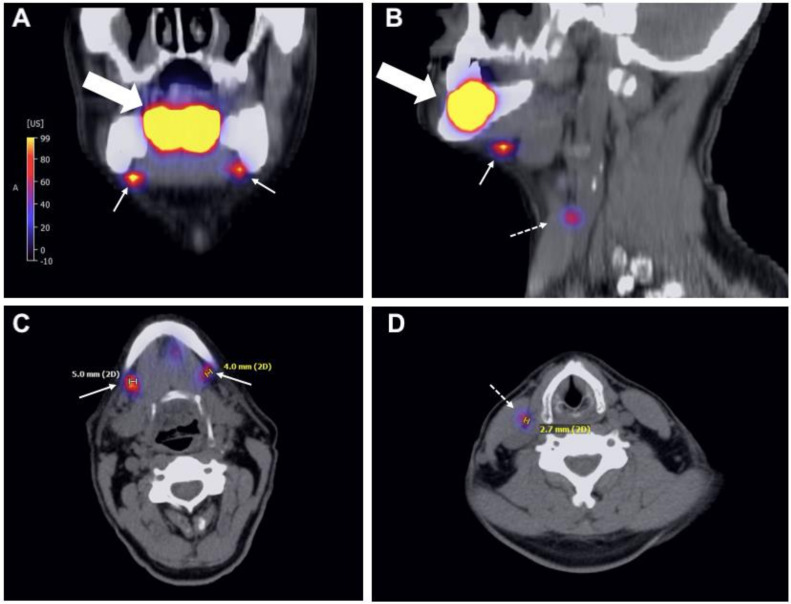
Multiplanar reconstruction of the SPECT/CT of patient no. 9: the two pictures above show the primary tumor of the floor of mouth (thick arrow) and the two foci in level Ib in the coronal (A) and sagittal planes (B). In the latter picture, a non-sentinel node downstream of the right sentinel node is marked with a thin dotted arrow. The pictures (C,D) show the two sentinel lymph nodes and one non-sentinel lymph node in the axial plane.
